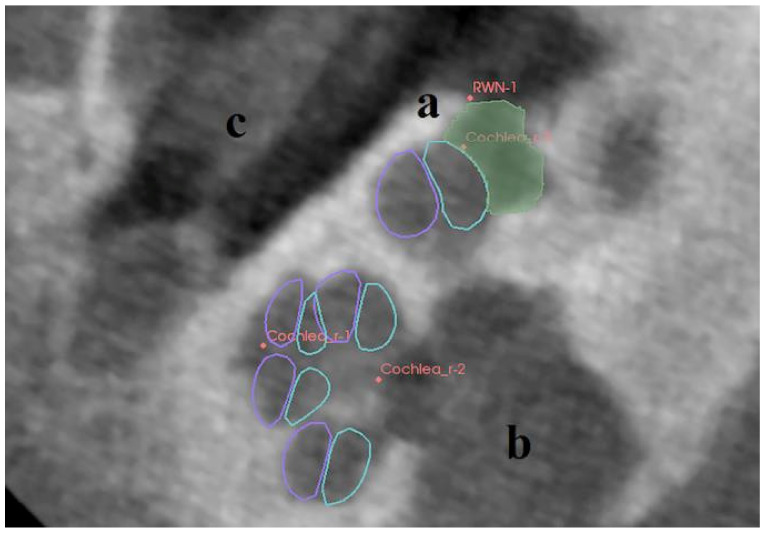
Using 3D Slicer and a custom made plug-in, points were placed manually at the midmodiolar apex (Cochlea_r-1), at midmodiolar basal turn (Cochlea_r-2), at the RWM (Cochlea_r-3) and on the tip of the bony overhang (RWN-1). These points allowed the determination of the midmodiolar axis by fitting a mean model of the ST (in turquoise) and the SV (in purple) in the CBCT data. The manually segmented RWN is shown in green. (a) bony overhang, (b) inner ear canal with N. vestibulocochlearis and N. facialis, (c) middle ear mucosa.
